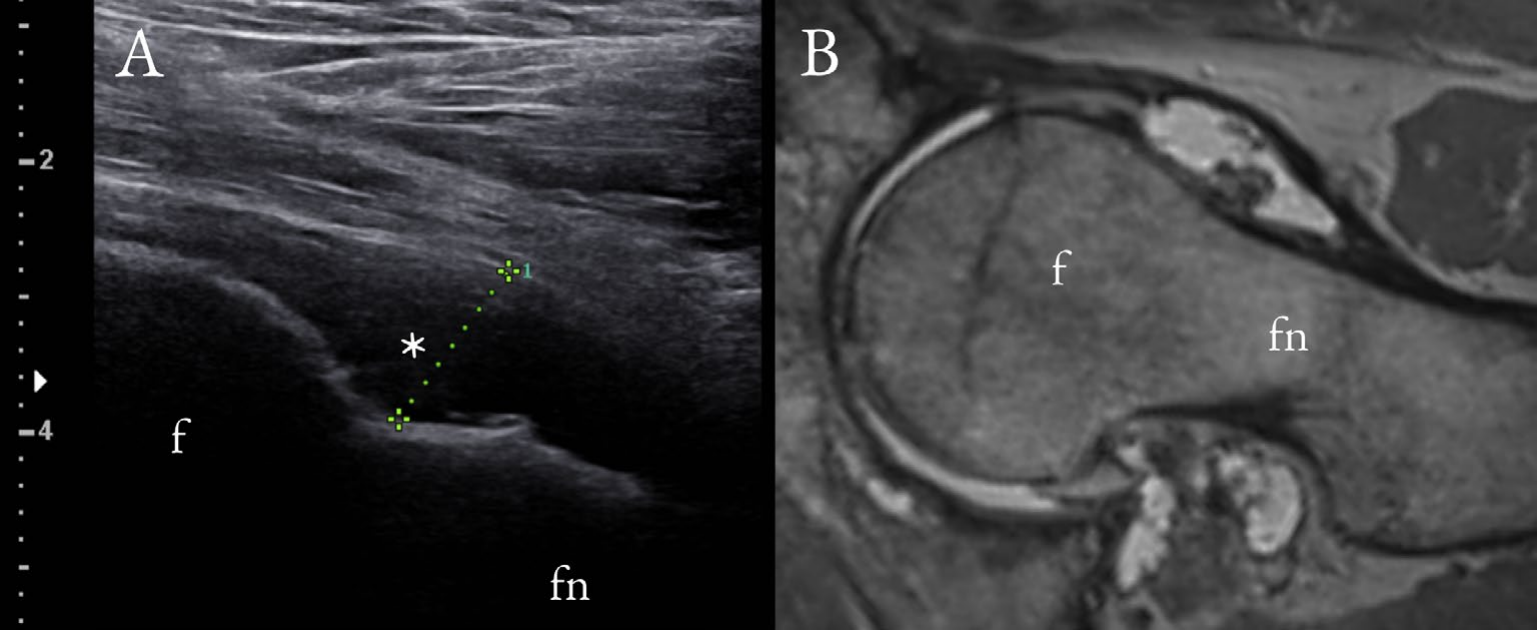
A 68‐year‐old man with moderate‐large hip joint effusion visualized in longitudinal US (asterisk) (A). PD‐weighed MRI sequence in oblique axial reformation (B) also shows clear hip effusion (black asterisk) as well as synovial hypertrophy, thought to be secondary to osteoarthritis. No evidence of hip effusion was seen in anteroposterior CR (not shown).
